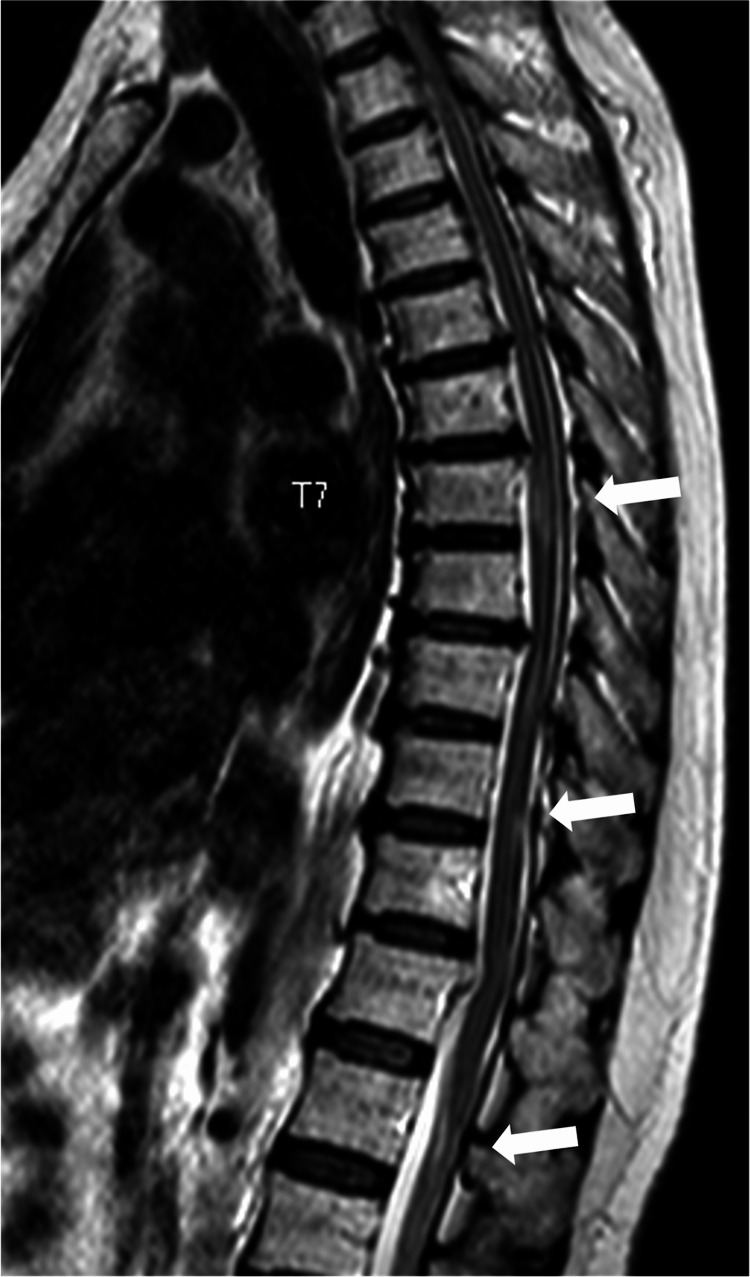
In the thoracic spine MRI, spinal canal stenosis was seen at Th5/6, Th6/7, and Th11/12. Intramedullary signal changes were seen in Th6, 7, 9, and 10/11(white arrow).
